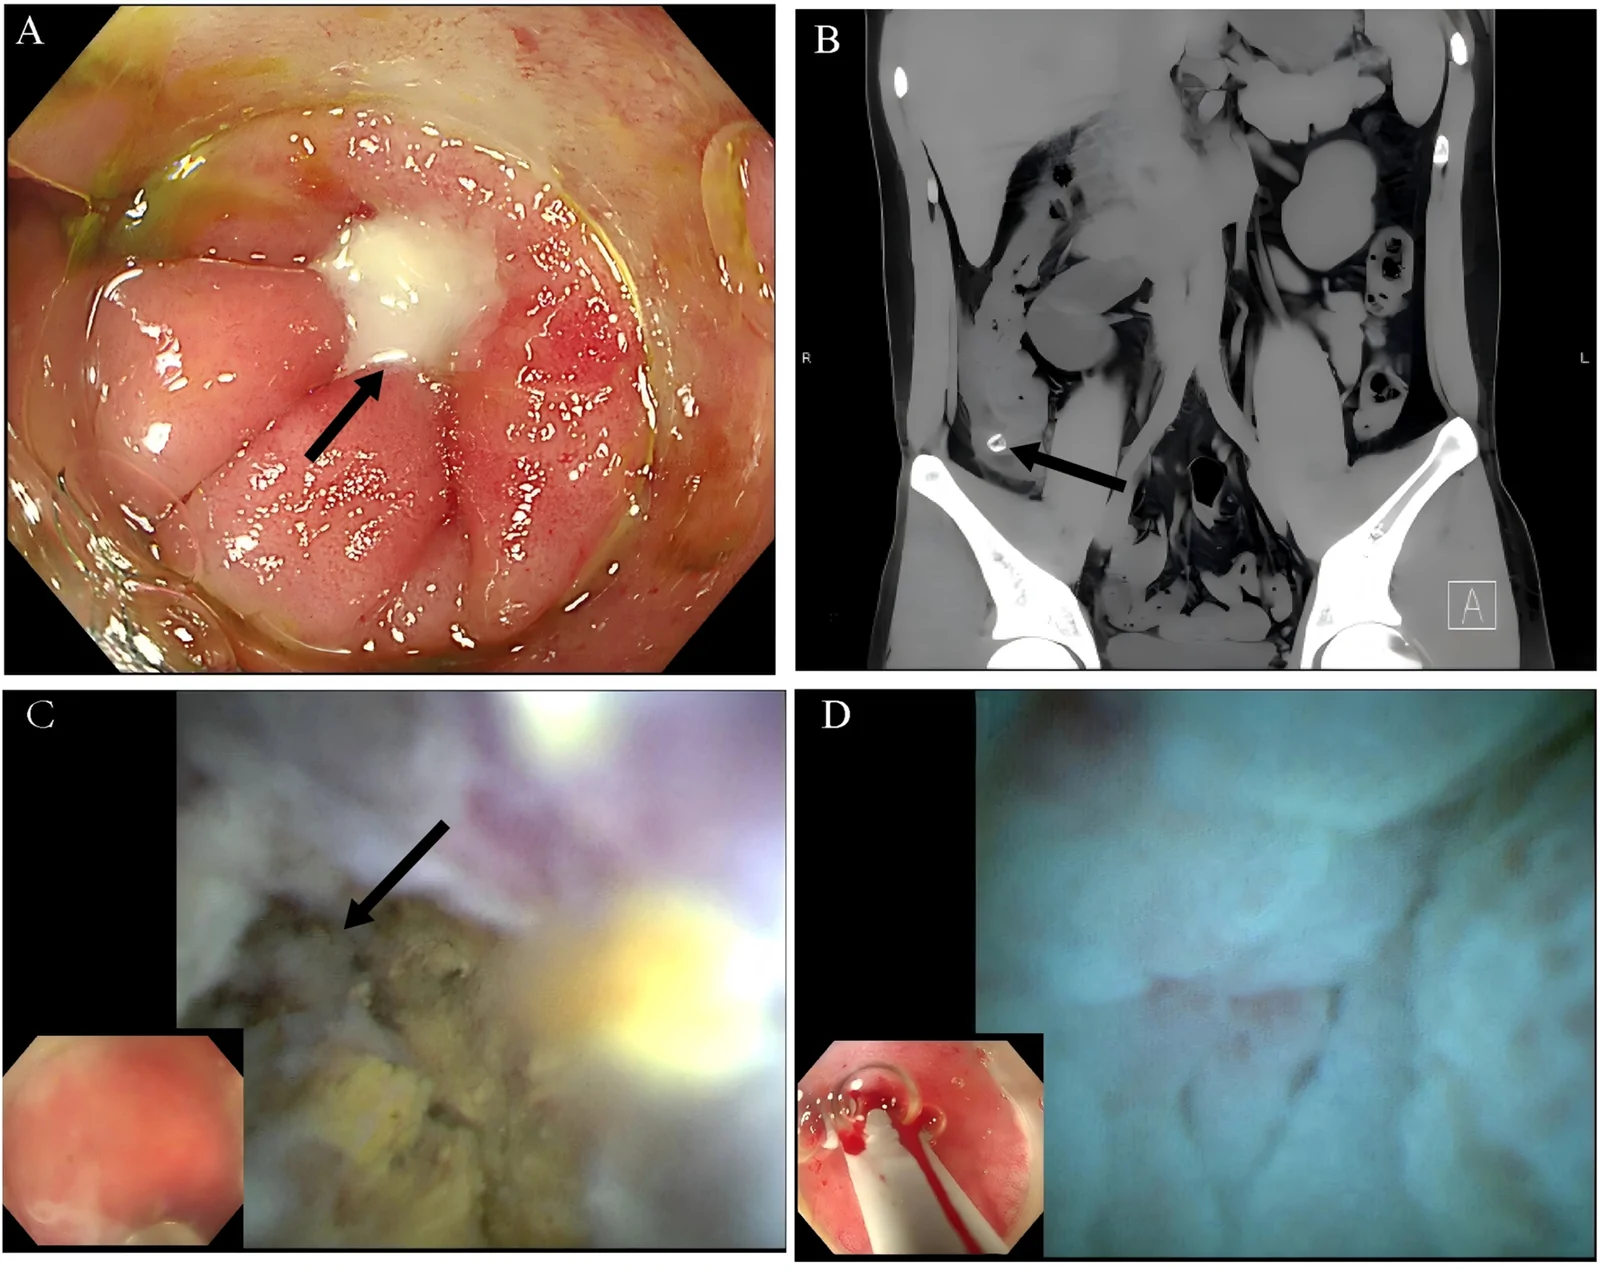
EyeMax-assisted direct visualization-guided intradiverticular intervention for inflammatory diverticular disease. (A) Conventional colonoscopy revealed spontaneous purulent discharge emerging from the diverticular orifice, suggesting active inflammatory diverticular disease. The arrow indicates purulent secretion from the diverticular opening. (B) Computed tomography demonstrated localized diverticulitis with inflammatory changes surrounding the affected diverticulum. The arrow indicates the inflamed diverticulum and associated intradiverticular fecalith. (C) EyeMax-assisted intradiverticular exploration revealed an impacted fecalith, edematous diverticular mucosa, and inflammatory debris within the diverticular cavity. The arrow indicates the retained fecalith. (D) Direct visualization-guided irrigation and endoscopic removal of inflammatory materials and fecalith were performed. Repeat EyeMax examination confirmed clearance of the diverticular cavity.
